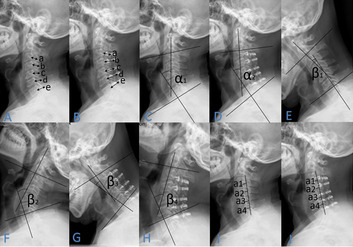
On the lateral radiograph of cervical spine, we measured the SCD of the C3-C7 segments and used the mean SCD [(a + b + c + d + e)/5] to evaluate the space of spinal canal before and after the operation (A, B), the C2–7Cobb Angle before and after the operation (C, D), the ROM before operation (β1 + β2) and the postoperative (β3 + β4) (E, F, G, H), the CCI before and after the operation, CCI = (a1 + a2 + a3 + a4)/A (I, J)
